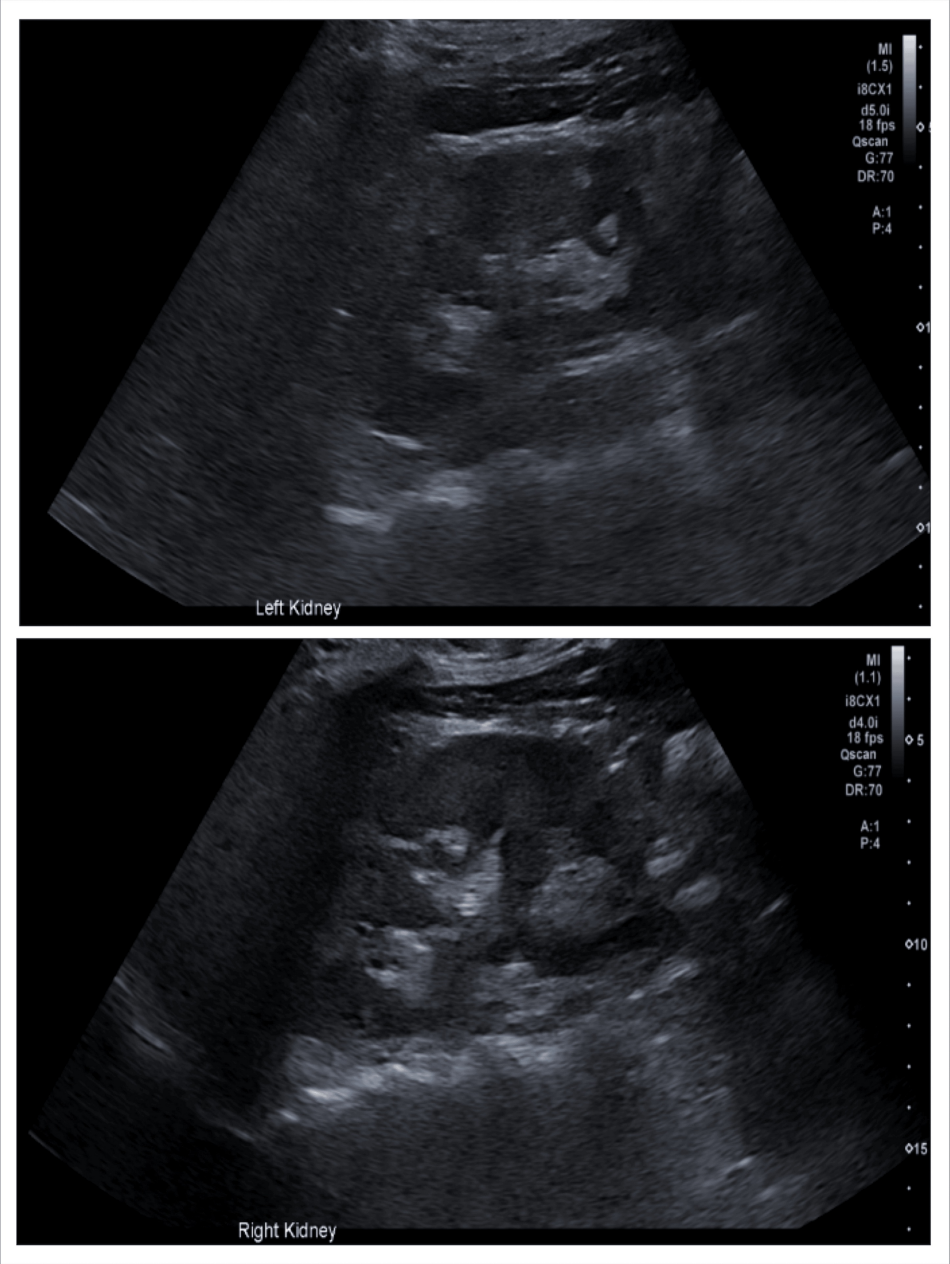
Ultrasound of kidneys showing the normal appearance of both kidneys
